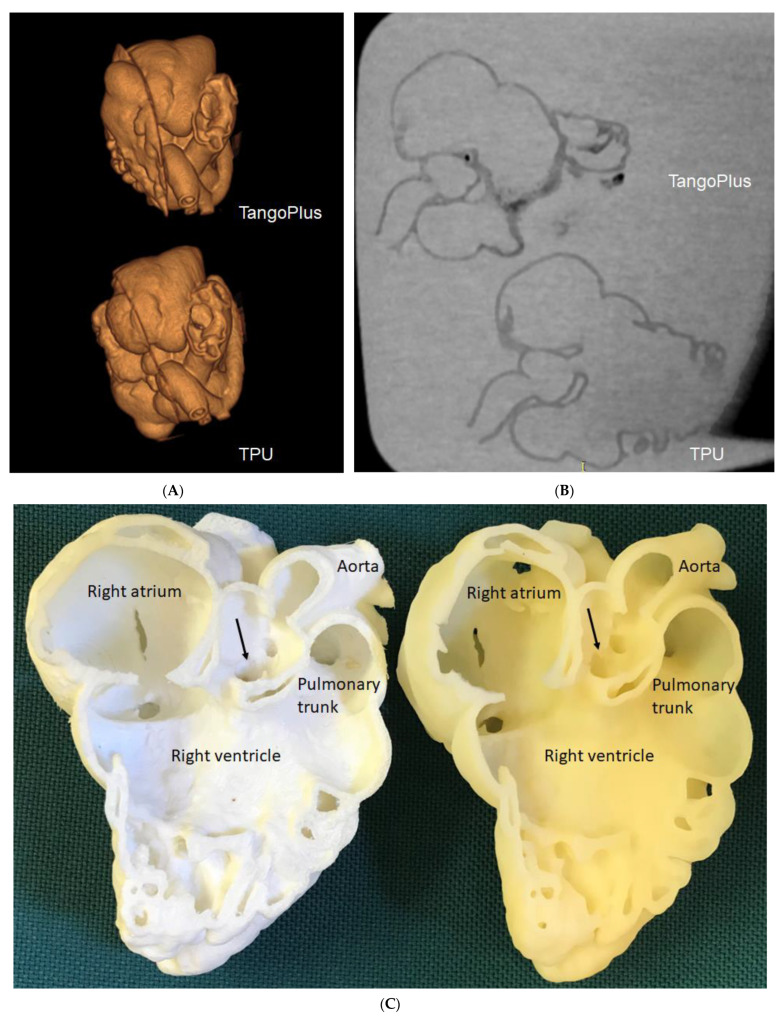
Three-dimensional-printed CHD models with the use of different materials for comparison of model accuracy. (A): Three-dimensional CT volume rendering of the 3D-printed models showing similar anatomical details. (B): Two-dimensional axial CT views of the 3D printed models. (C): Inside view of cardiac chambers and aortic structures on both models. The white model is printed with TPU, while the yellow model is printed with TangoPlus. Arrows refer to the subaortic ventricular septal defect.
